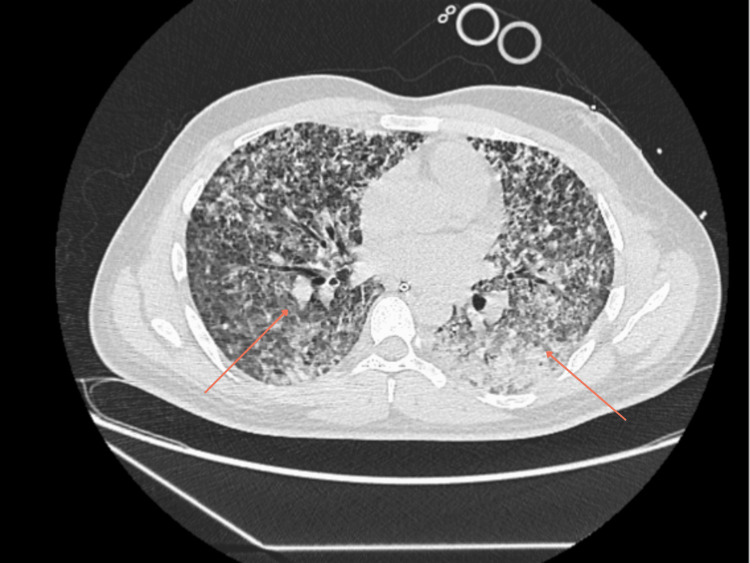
High Resolution CT Thorax Demonstrates extensive bilateral dense airspace shadowing, which is worse in the lower lobes, especially on the left. No pulmonary haemorrhage seen.
